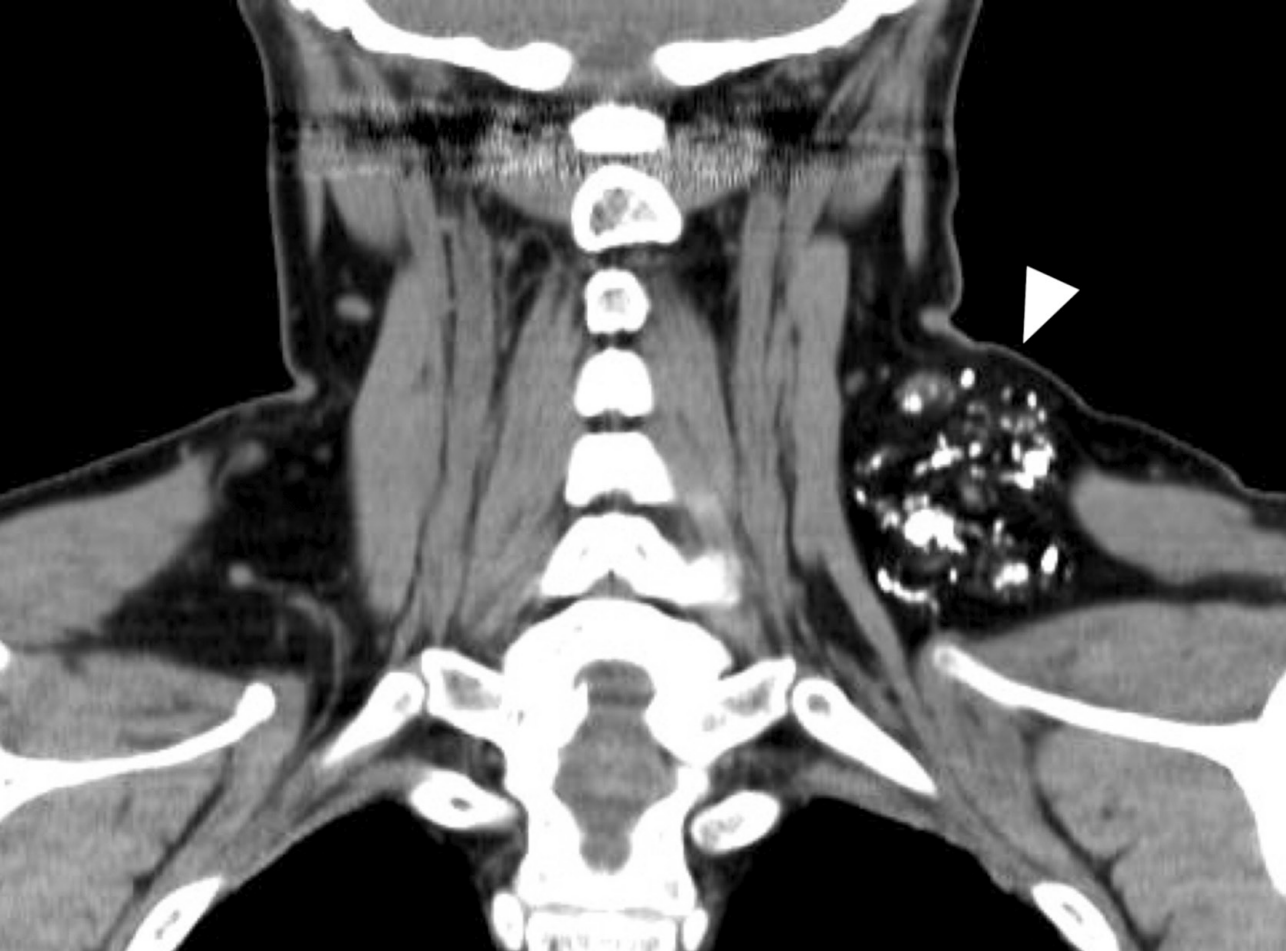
Computed tomography scan obtained after intranodal lymphangiography showing a hyperabsorptive zone within the lesion (white arrowhead) that was thought to represent lipiodol deposition.
